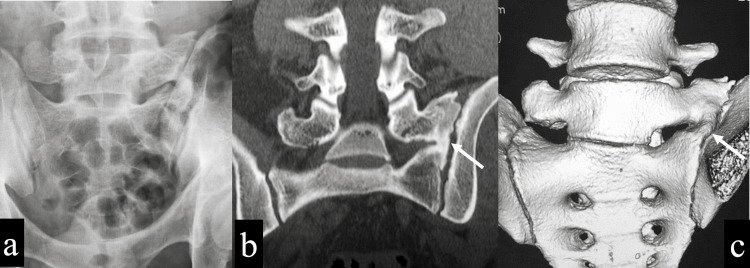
Imaging findings two years after surgery a: Plain anteroposterior X-ray showed further bony regeneration around the transverse process. b: CT coronal view showed bony continuity of the transverse process and sacral alar (white arrow). c: CT 3D image showed bony continuity of the transverse process and sacral alar (white arrow).
